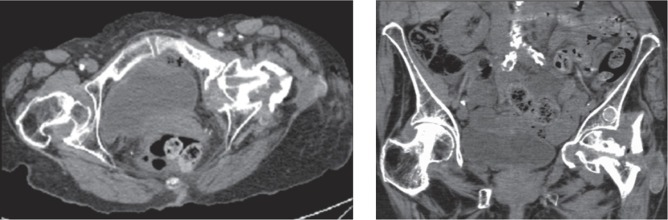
CT scans showing the canal through which the hip pin had migrated through the femoral head and the pelvic bone before entering the abdomen.
